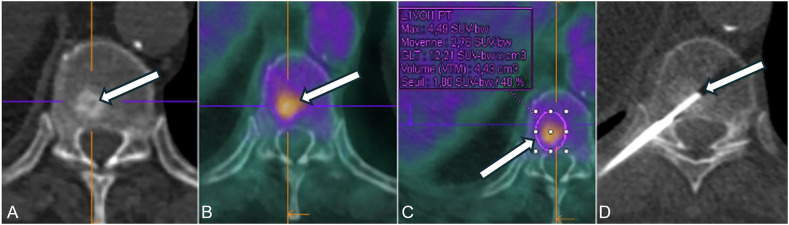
A 68-year-old patient with a history of bronchogenic carcinoma, surgically treated in 2023. The following imaging revealed a hypermetabolic sclerotic lesion at T10. A An uninjected CT presented in axial section corresponding to the vertebrae. B PET/CT with hypermetabolic vertebral lesion. C PET/CT with region of interest and metabolic parameters. D CT-guided core biopsy of vertebral lesion, with needle and trephine
